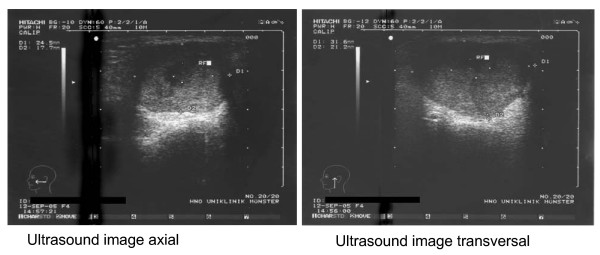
Pleomorphic Adenoma of the left parotid gland: Ultrasound image axial and Ultrasound image transversal
